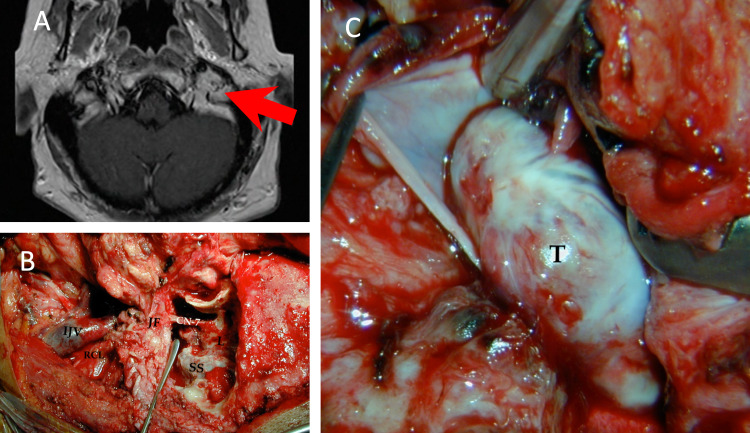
Glomus jugulare tumor accessed via jugular triangle. Exposure of the deeper surgical triangles, including the jugular triangle. (A) Axial T1 MRI scan demonstrating a left glomus jugulare tumor in a patient in their mid-40s presenting with hearing loss and pulsatile tinnitus (case 9). A lateral distal cervical retro-auricular transtemporal (infra-labyrinthine) approach was performed to expose the jugular foramen (JF) (B). The fallopian canal was skeletonized keeping a bone shell around the facial nerve (CN-7) to preserve it. After ligating the sigmoid sinus and the jugular vein, the vein was opened, and the tumor resected from within (C). The medial wall of the vein was preserved to protect the neighboring lower cranial nerves. Labels: CN-7: facial nerve; IJV: internal jugular vein; JF: jugular foramen; L: labyrinth; RCL: rectus capitis lateralis; SS: sigmoid sinus.
